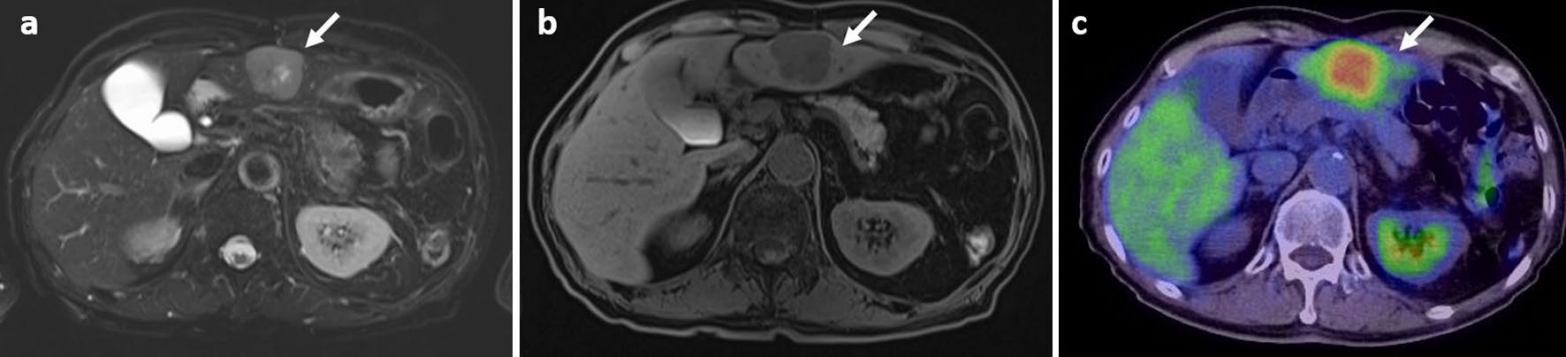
a–c Magnetic resonance imaging (MRI) and computed tomography: a fat-saturation T1-weighted image; b fat-saturation T2-weighted image; and c combined positron emission tomography and computed tomography (PET–CT). MRI showing a large mass in the left lateral segment of the liver, which is indicated by a low-intensity signal on T1-weighted images and a high-intensity signal on T2-weighted images and DWI. PET–CT showing that the maximum standardized uptake value of the tumor in the left lateral segment of the liver was 12.6
